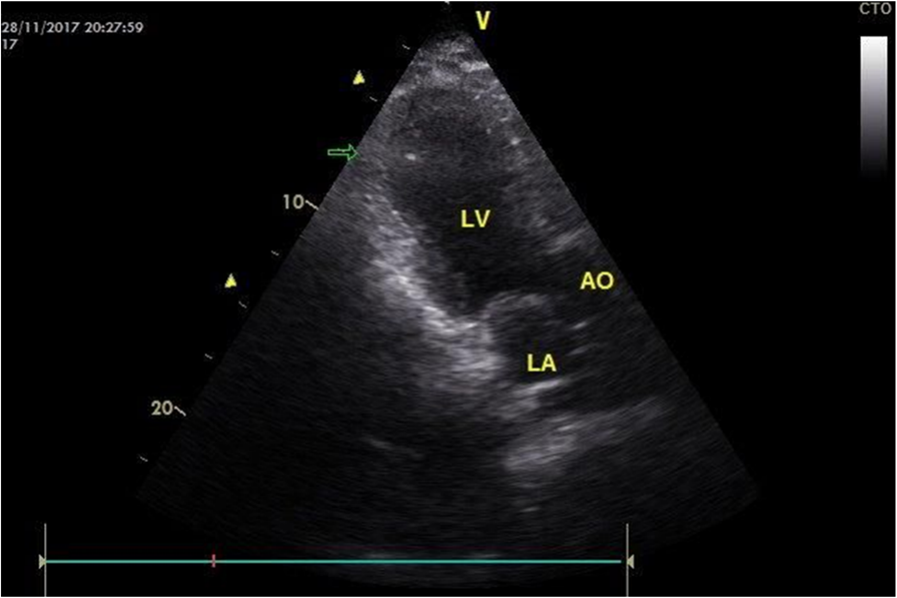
Transthoracic echocardiography. Endsystolic apical five-chamber view on hospital day 1 showing dyskinesia of the left ventricular posterior, posterolateral and apical parts. *AO* aorta, *LA* left atrium, *LV* left ventricle
